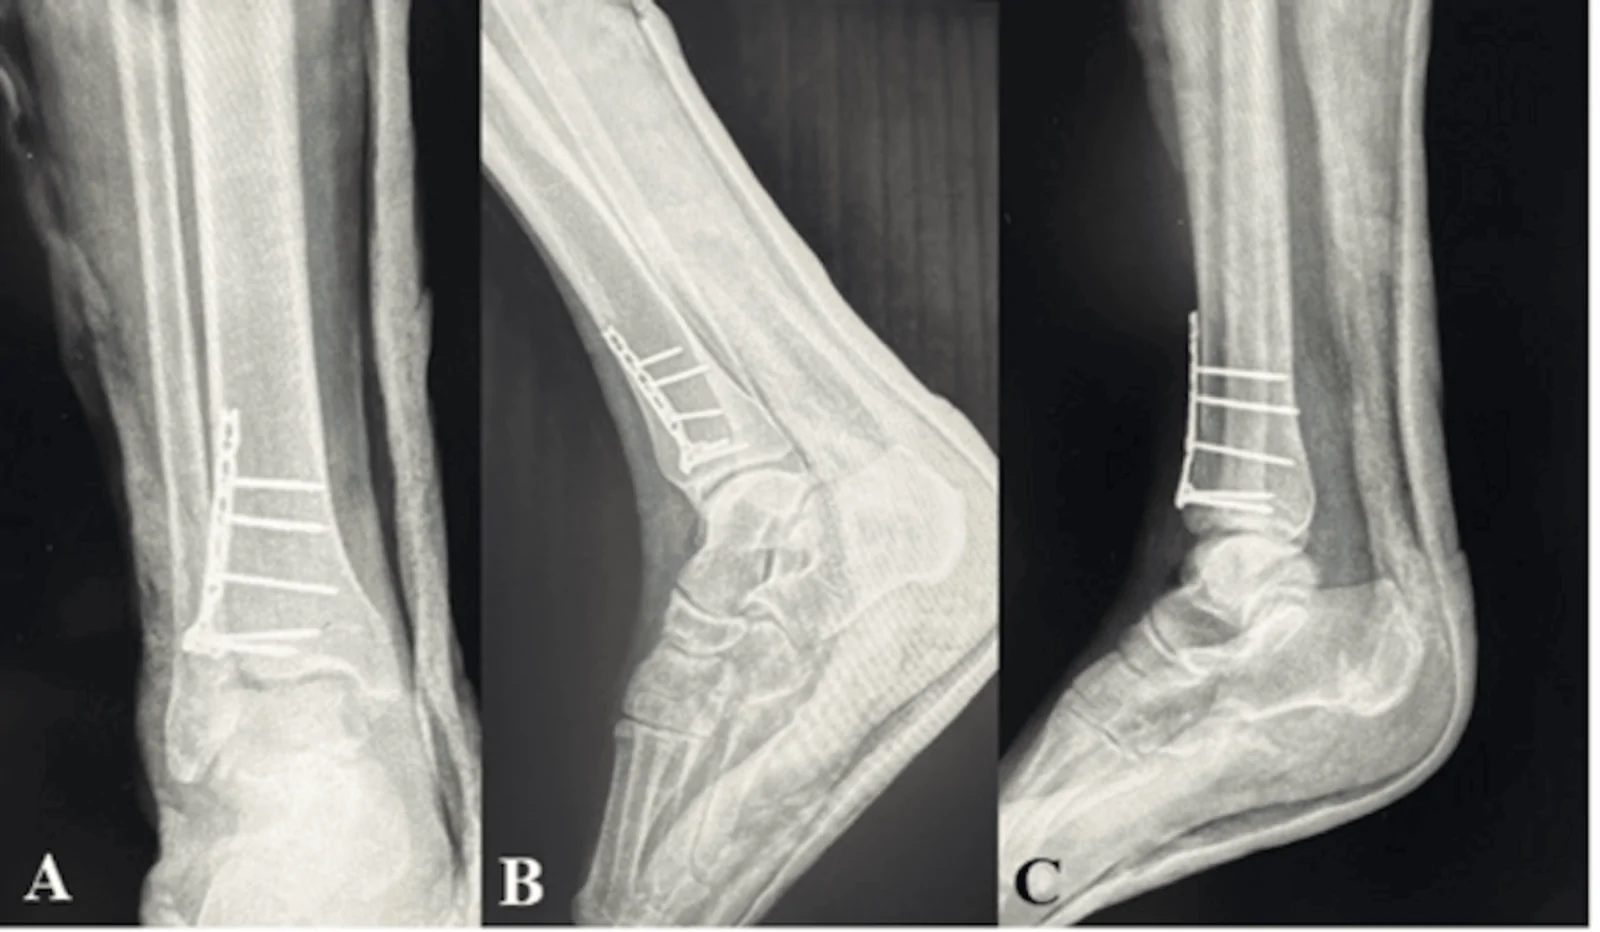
Initial ankle postoperative X-rays. Initial ankle postoperative anteroposterior (A), lateral (B) and external rotation (C) X-ray views showing the excellent reduction of the Chaput fragment, the final restoration of the joint congruency and alignment, and the final position of the plate.
